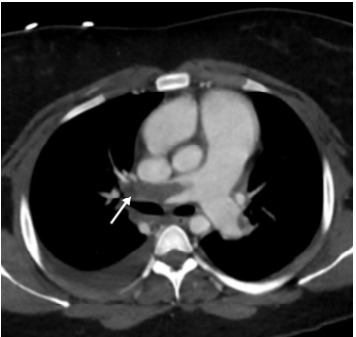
Near-complete filling defect of the right main pulmonary artery consistent with pulmonary embolism seen on computed tomography of the chest and denoted by white arrow.
